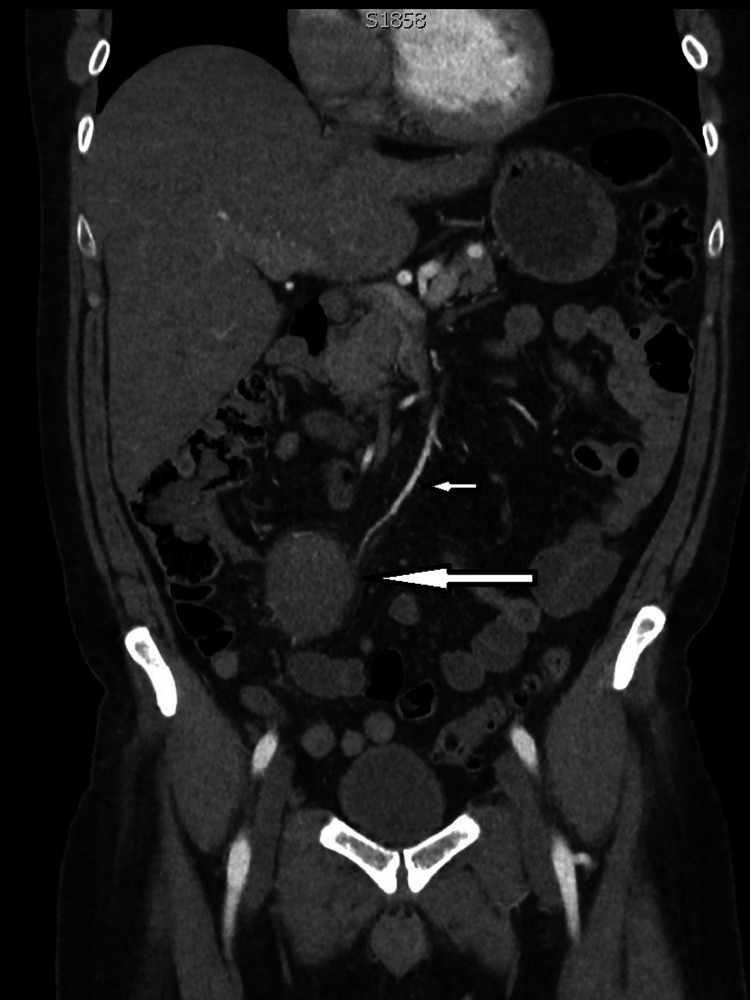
Coronal image in arterial phase of CT abdomen and pelvis which shows the feeding vessel (small arrow) into the pseudoaneurysm (big arrow) which is arising from a terminal branch of SMA. SMA, superior mesenteric artery
